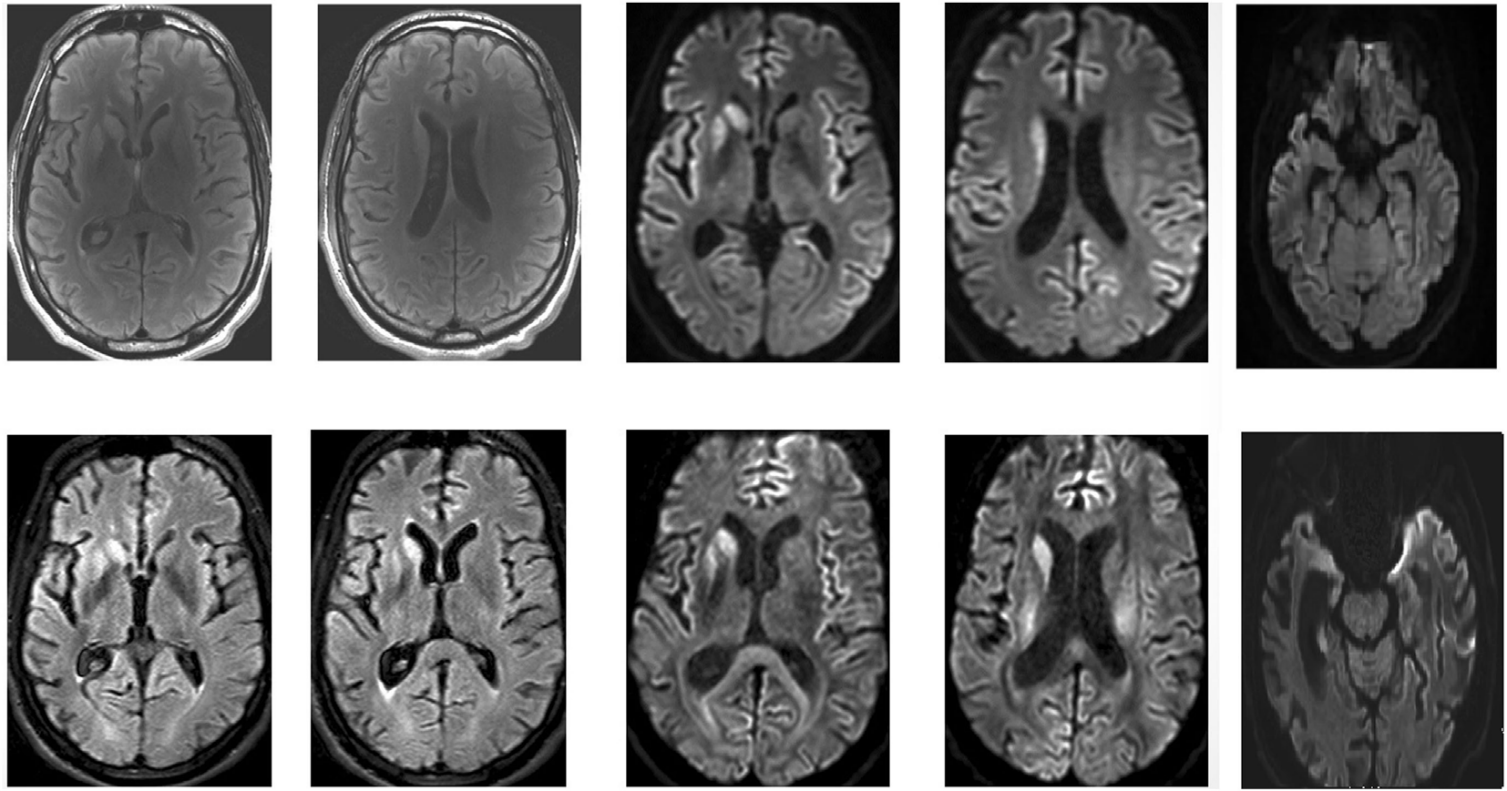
Magnetic resonance imaging of the patient’s brain. Top: April 2016. Bottom: May 2016. Asymmetric T2, fluid-attenuation inversion recovery (FLAIR), and diffusion-weighted (DWI) hyperintensities in the putamen and caudate, as well as frontal and temporal cortical regions of restricted diffusion are clearly visible.
